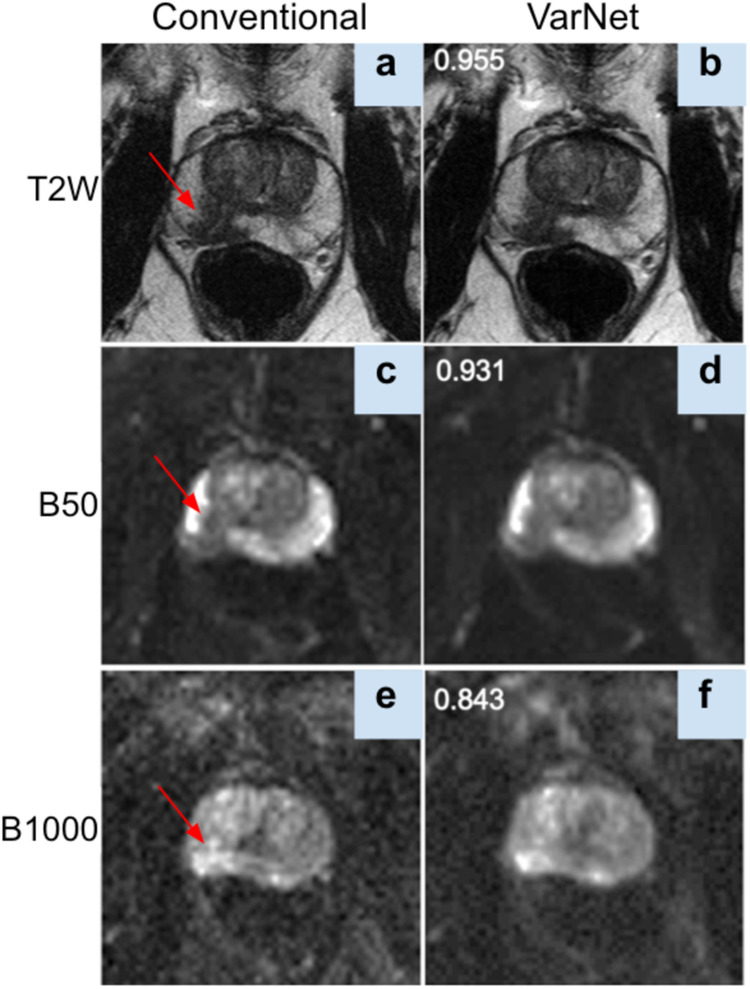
Representative example from the test set from the VarNet reconstruction with an effective acceleration R = 6 for T2-weighted (a,b) and R = 3 for the B50 (c,d) and B1000 (e,f) images. Red arrows indicate a lesion in the right posteromedial midgland peripheral zone, which is visible in both the conventional and accelerated reconstructed images. Numbers in images b-f correspond to SSIM values between the image and its conventional reconstruction counterpart.
